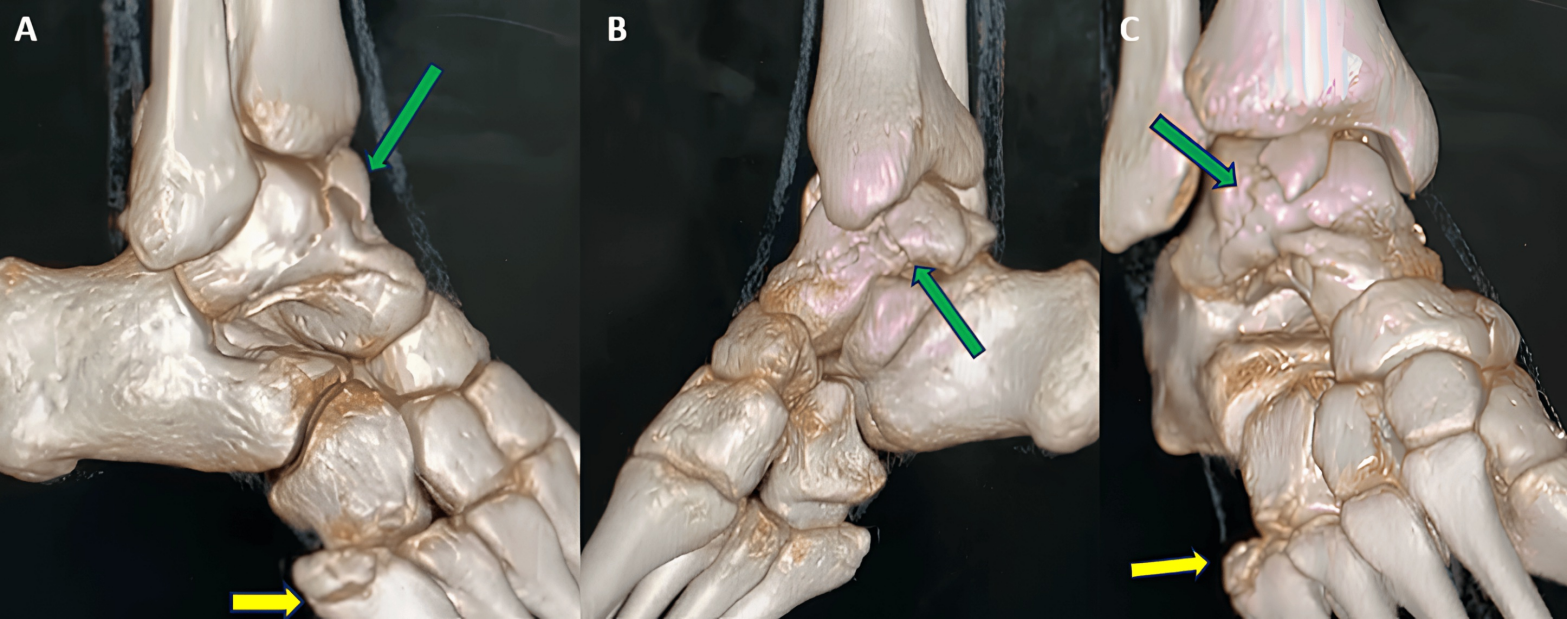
CT 3D images of the right ankle and right foot. The images show (A) lateral view of right ankle showing talus fracture extension into head, neck, subtalar joint, and displaced osteochondral fracture fragment (green arrow), fifth metatarsal base fracture (yellow arrow), (B) medial view of right ankle showing talus fracture extension into head, neck, subtalar joint (green arrow), fifth metatarsal base fracture (yellow arrow), and (C) anteroposterior view of right ankle showing talus fracture extension into head, dome, neck of talus and displaced osteochondral fracture fragment (green arrow), fifth metatarsal base fracture (yellow arrow).
